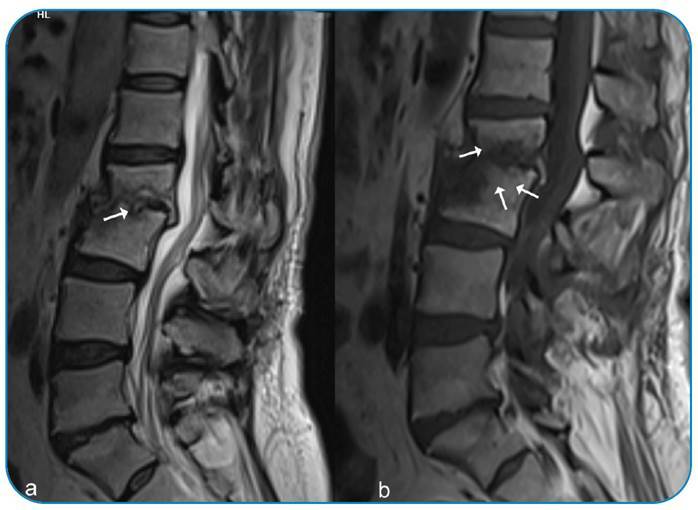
Posttreatment MRI scan. (a) The sagittal T2-weighted image without fat suppression shows persisting erosions in the vertebral endplates (arrow) but resolution of the hyperintense signals in the disc space and epidural area. (b) The sagittal T1-weighted image without fat suppression shows decreased signal losses (arrows) in the vertebral bodies and disc space.
